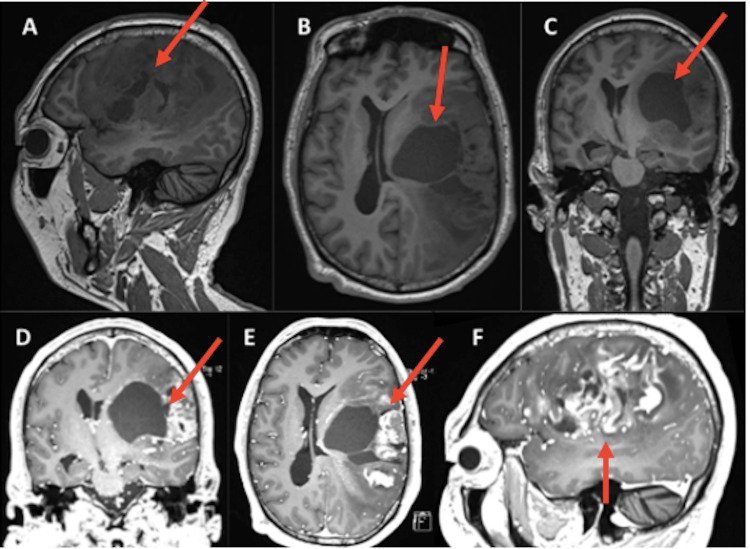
T1-weighted cranial MRI (A-C) and contrasted cranial MRI (D-F) A: sagittal section, diffuse tumor lesion in frontoparietotemporal region, heterogeneous, poorly defined borders, with central necrosis. B: axial section, tumor lesion with large cystic lesion corresponding to necrosis that displaces the midline structures, an insular component is observed. C, D: coronal section. E: axial section. F: sagittal section. Central necrosis is observed, with heterogeneous enhancement to the periphery.
